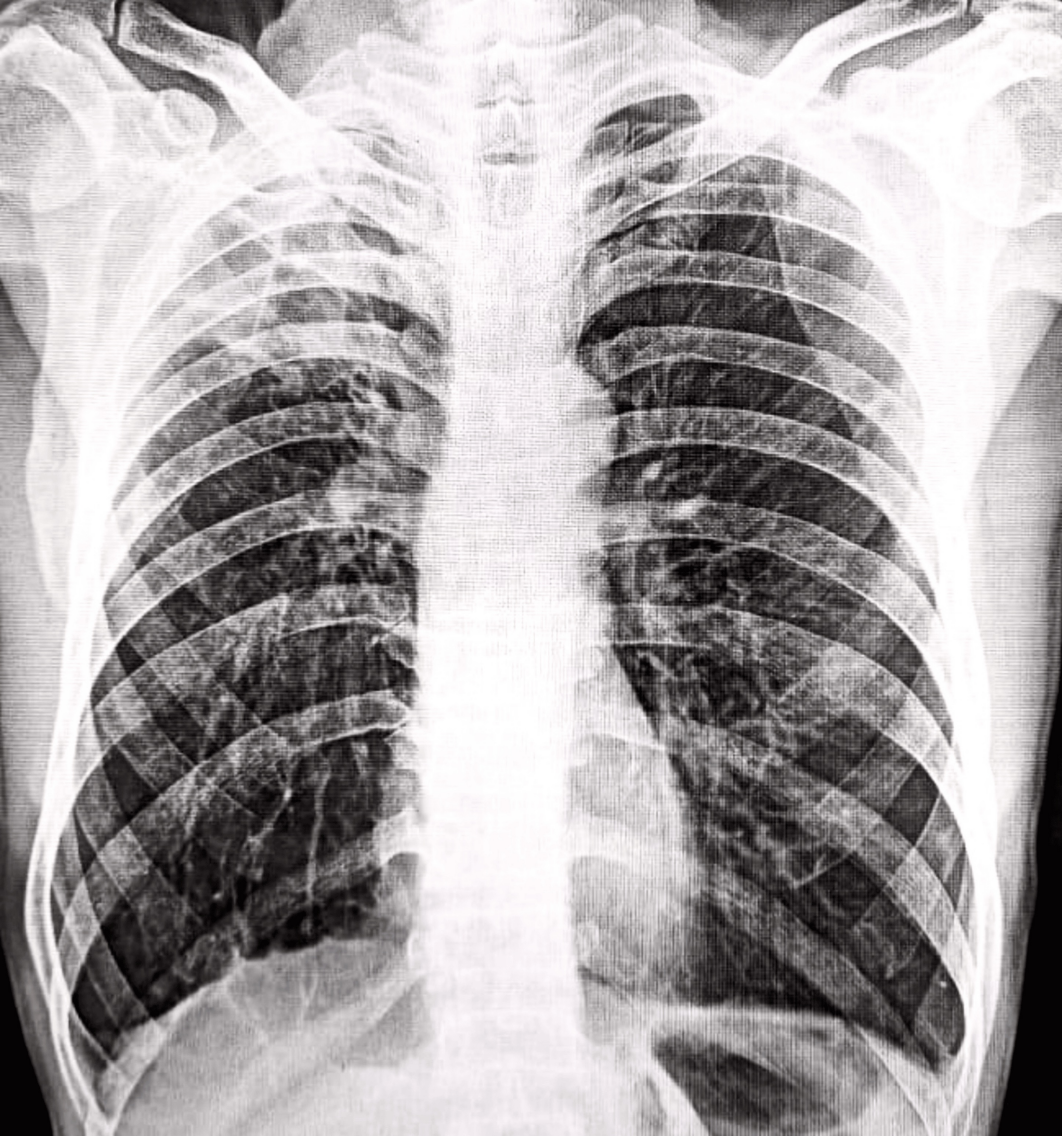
Post-procedure chest X-ray following bronchial artery embolization.
